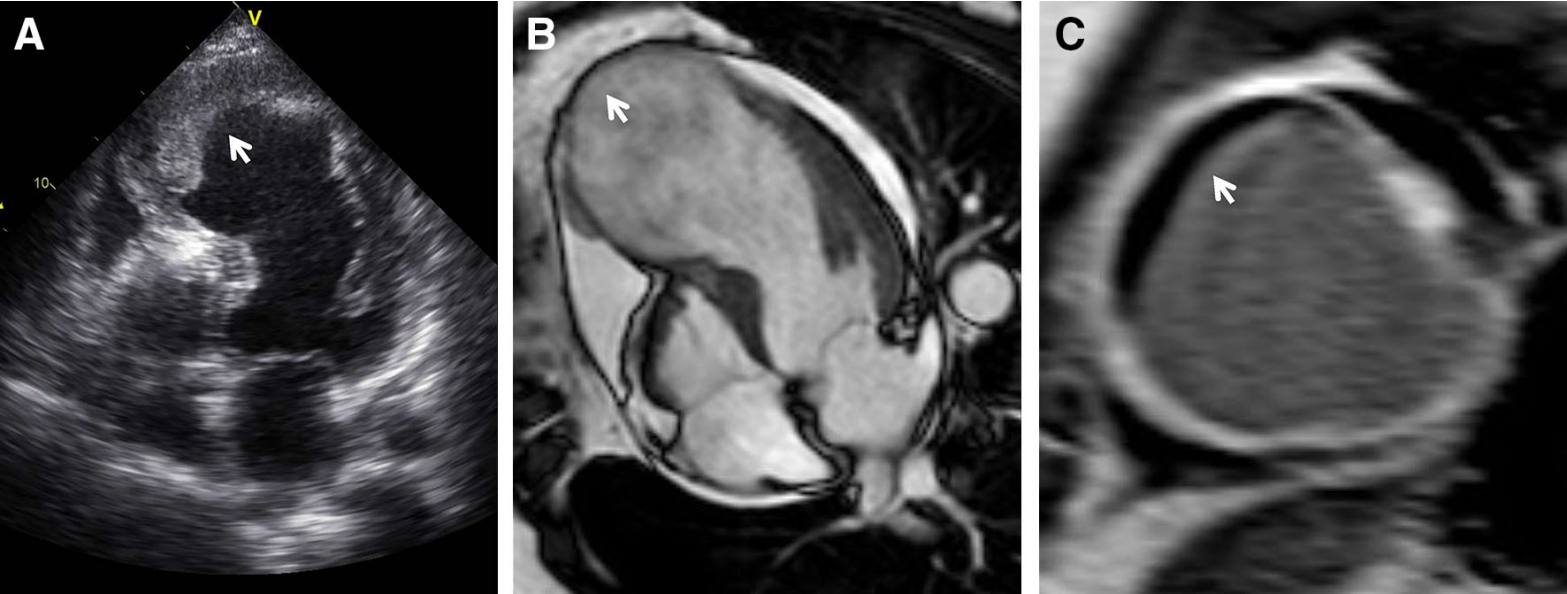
Example of a patient with large anterior myocardial infarction and subsequent formation of a large apical aneurysm. On transthoracic 2D echocardiography (A), the *arrow points* to the large apical aneurysm with thrombus formation. Magnetic resonance imaging shows a thin-walled apical aneurysm (*arrow*) extending from the mid septum to the apical lateral wall (B). On contrast-enhanced MRI (C), the short-axis view of the aneurysm shows hyperenhanced transmural scar (*white*) with a large apical thrombus (*black*, *arrow*)
